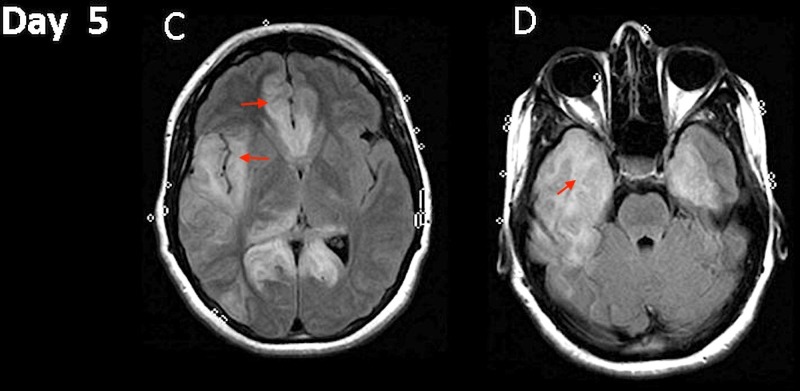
Magnetic resonance imaging (MRI). Hospital day 5 fluid attenuated inversion recovery (FLAIR) changes evolution (C and D).
